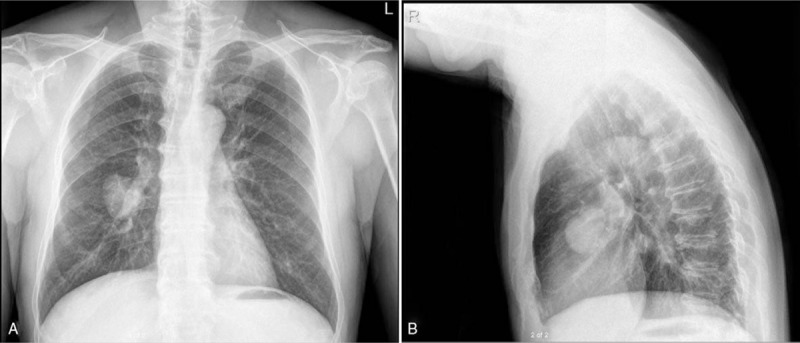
Images of chest forward (A) and lateral (B) digital radiography. It revealed bilateral thoracic symmetry and a mass with a diameter of about 5.2 cm was seen in the right middle lobe.
